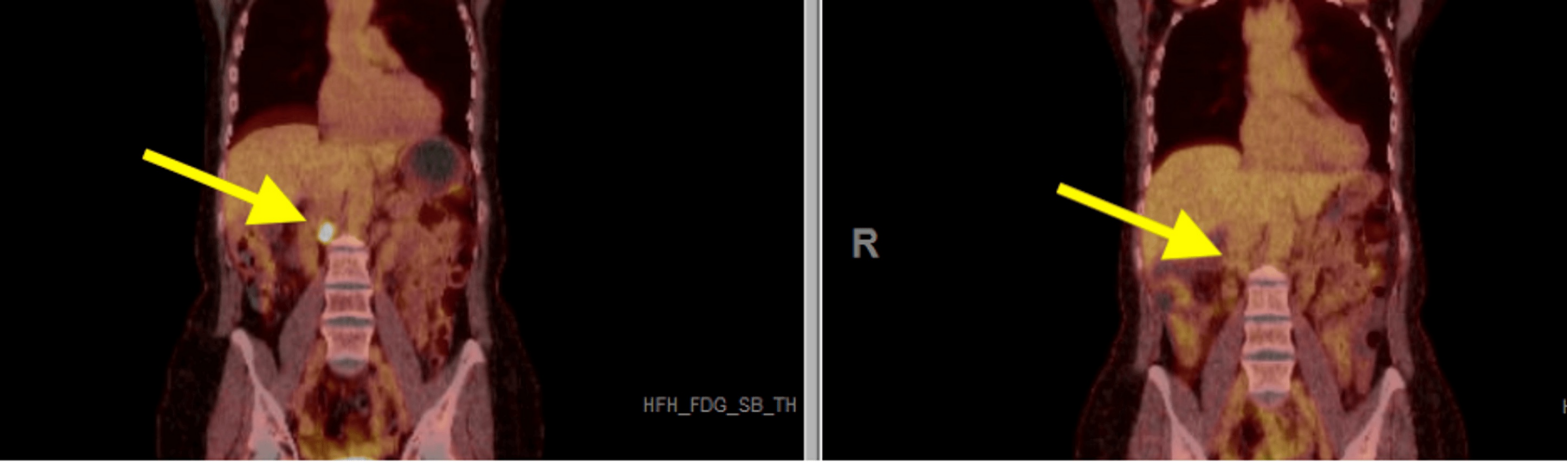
Comparison of PET-CT scans over six months of systemic therapy demonstrates near-complete resolution of the metastatic right adrenal lesion. PET-CT: Positron Emission Tomography Computed Tomography
